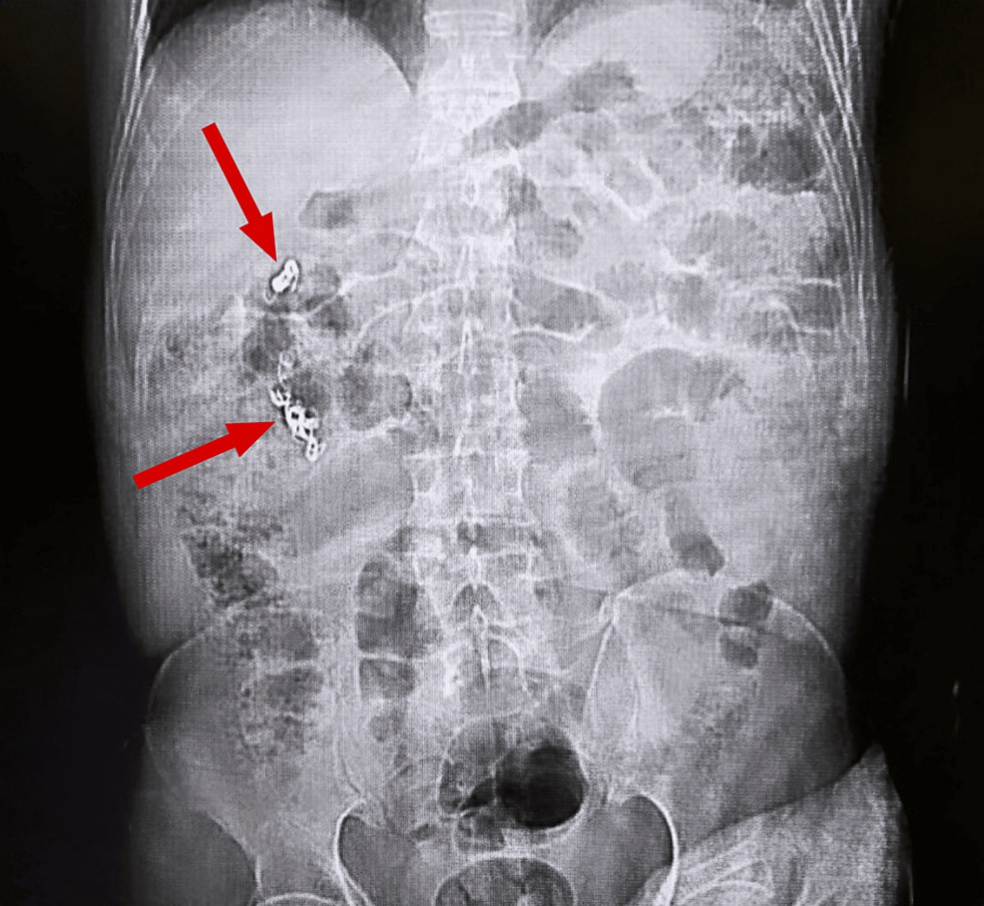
A KUB radiograph showing right renal vessel coils KUB, kidney, ureter and bladder
